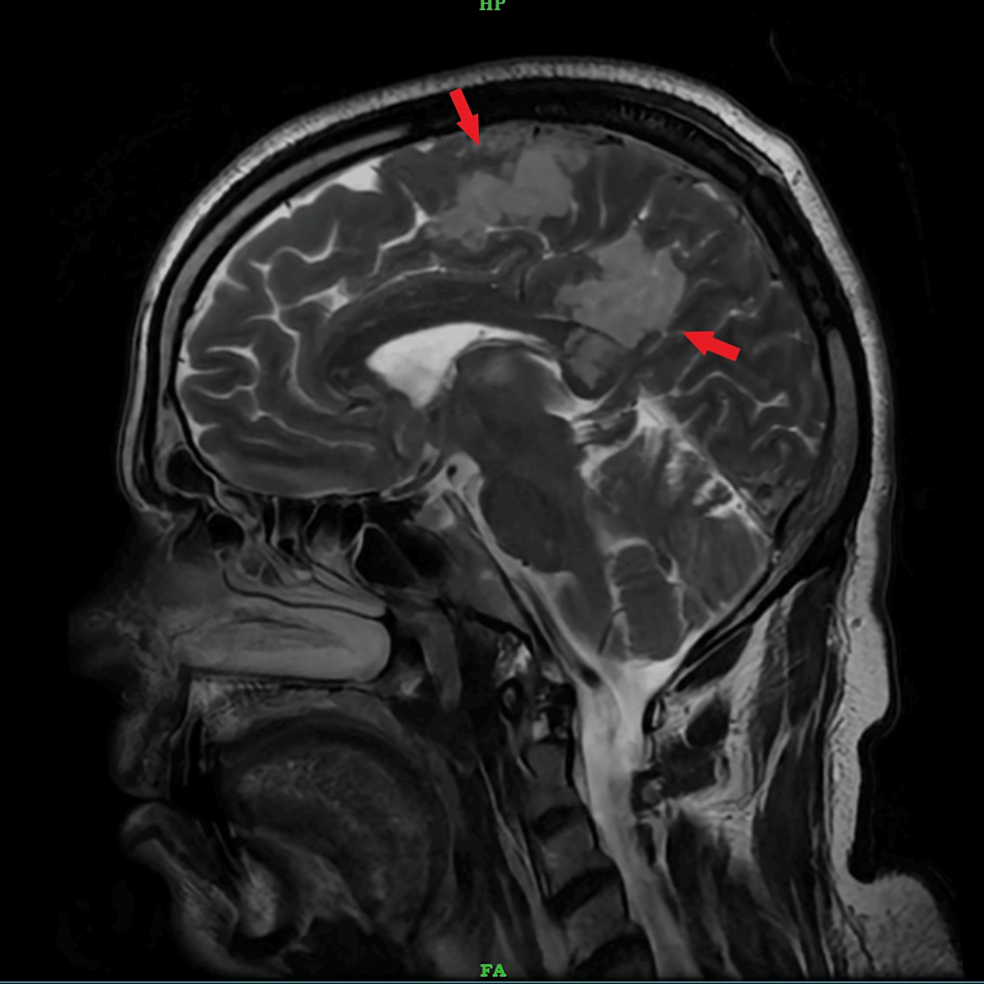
MRI brain T2-weighted sagittal section The red arrows indicate hyperintensities in the frontal lobe, cingulate gyrus, and splenium of the corpus callosum.
